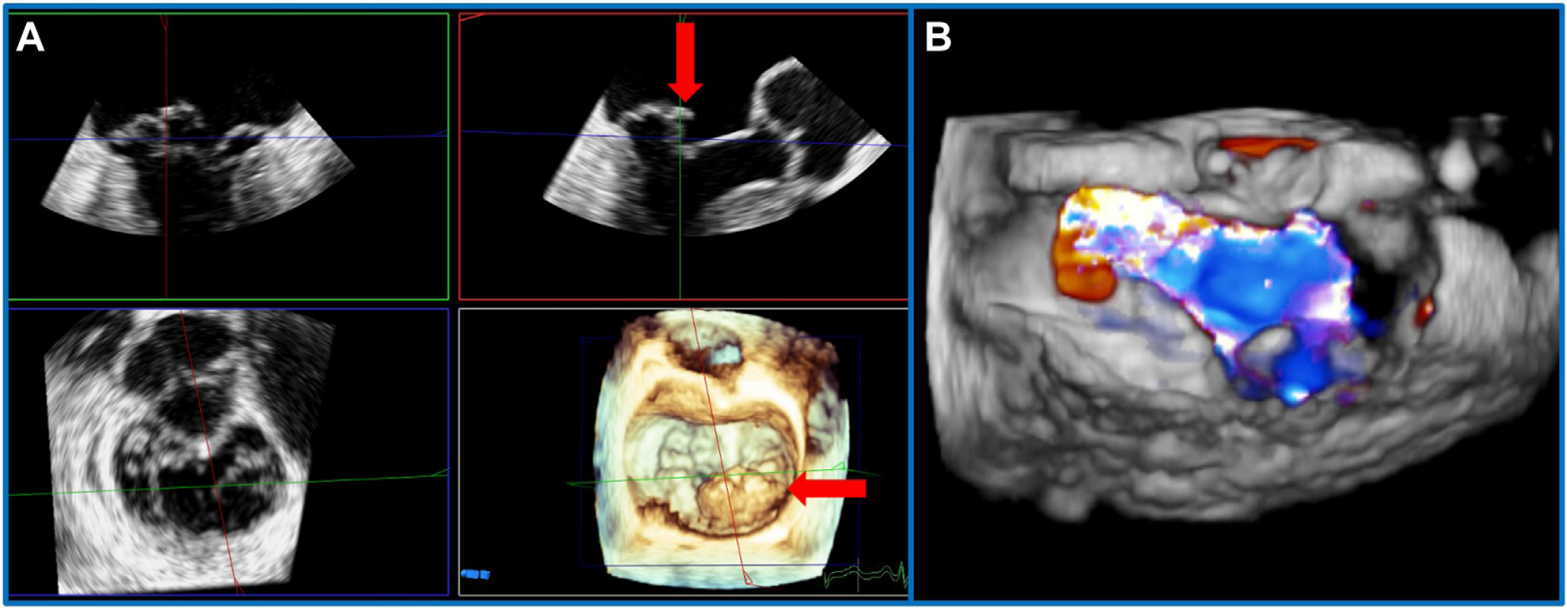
(A) Three-dimensional TEE, midesophageal, RT-MPR simultaneous orthogonal long-axis *(top)* and short-axis *(bottom)* 2D and volume-rendered reconstruction en face MV views, demonstrates extensive P_2_/P_3_ prolapse and isolated flail segment *(arrows)*. (B) Three-dimensional TEE, midesophageal, volume-rendered reconstruction en face MV view with color flow Doppler, demonstrates severe anteriorly directed MR.
